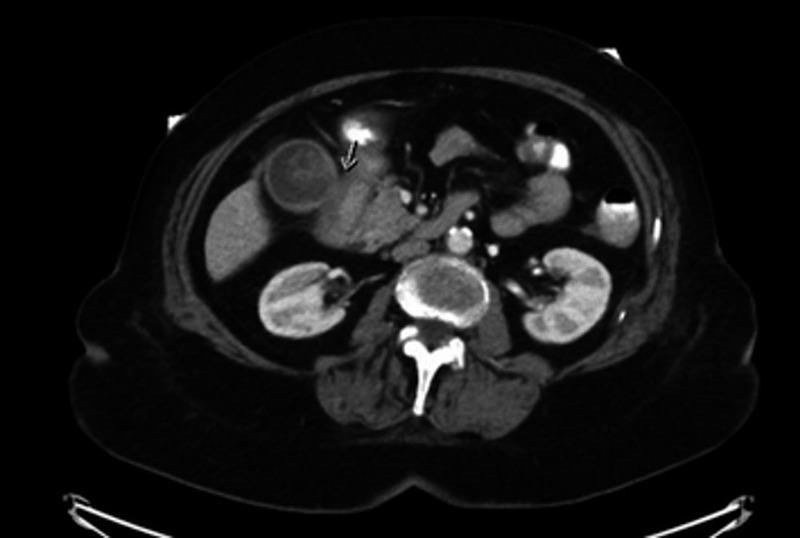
Abdominal computed tomography showing inflammatory changes with mural thickening of the duodenal wall
